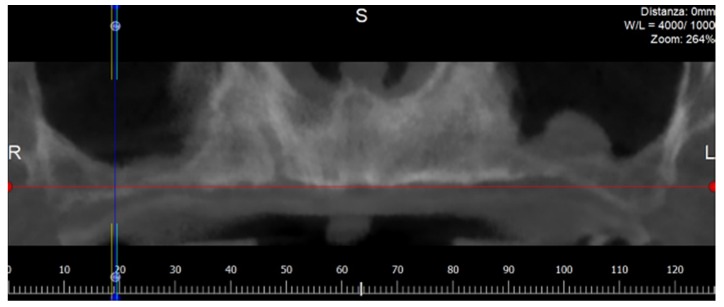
Panorex image elaborated by the software from a maxillary Cone Beam Computed Tomography (CBCT). L: Left; R: Right; S: Supper; L: Lower.
